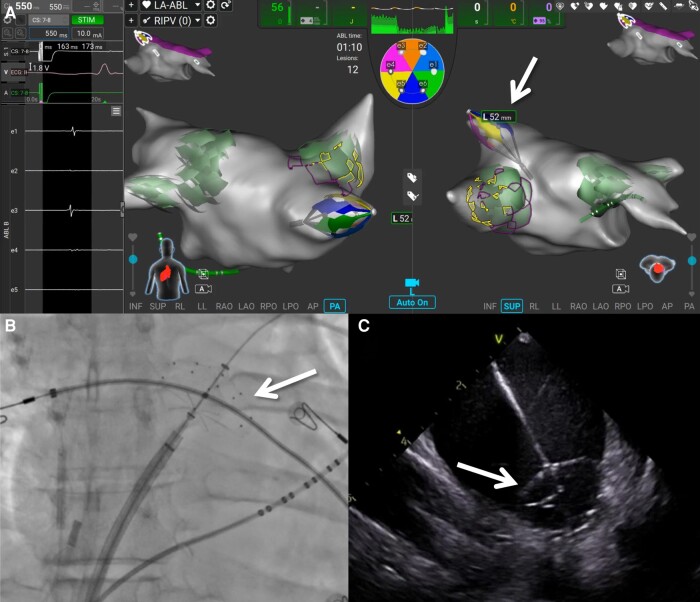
Pulmonary vein isolation using the PFA catheter. (*A*) A screenshot of the electroanatomical mapping system is shown with shadows (green shadows) of where the PFA lesions were placed, and the location of the ablation catheter in the right inferior pulmonary vein (white arrows) in both posterior (left) and superior (right) views. The PFA catheter (white arrows) is also shown at the left superior pulmonary vein antrum in a large basket configuration on fluoroscopy (*B*) and on intracardiac echocardiography (*C*). While not formally analysed, PVs were typically acutely isolated with the first PF application; subsequent successively proximal applications extended the level of isolation and acted as bonus lesions to enhance durability. See online Supplementary material online, *Video S2* for an example of PFA of a PV.
